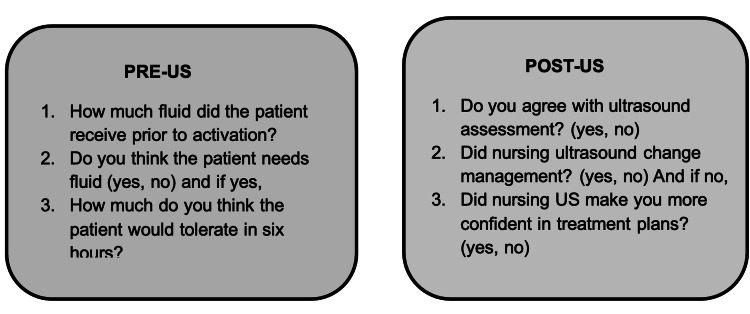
Pre- and post-ultrasound assessment surveys for physicians US = Ultrasound
